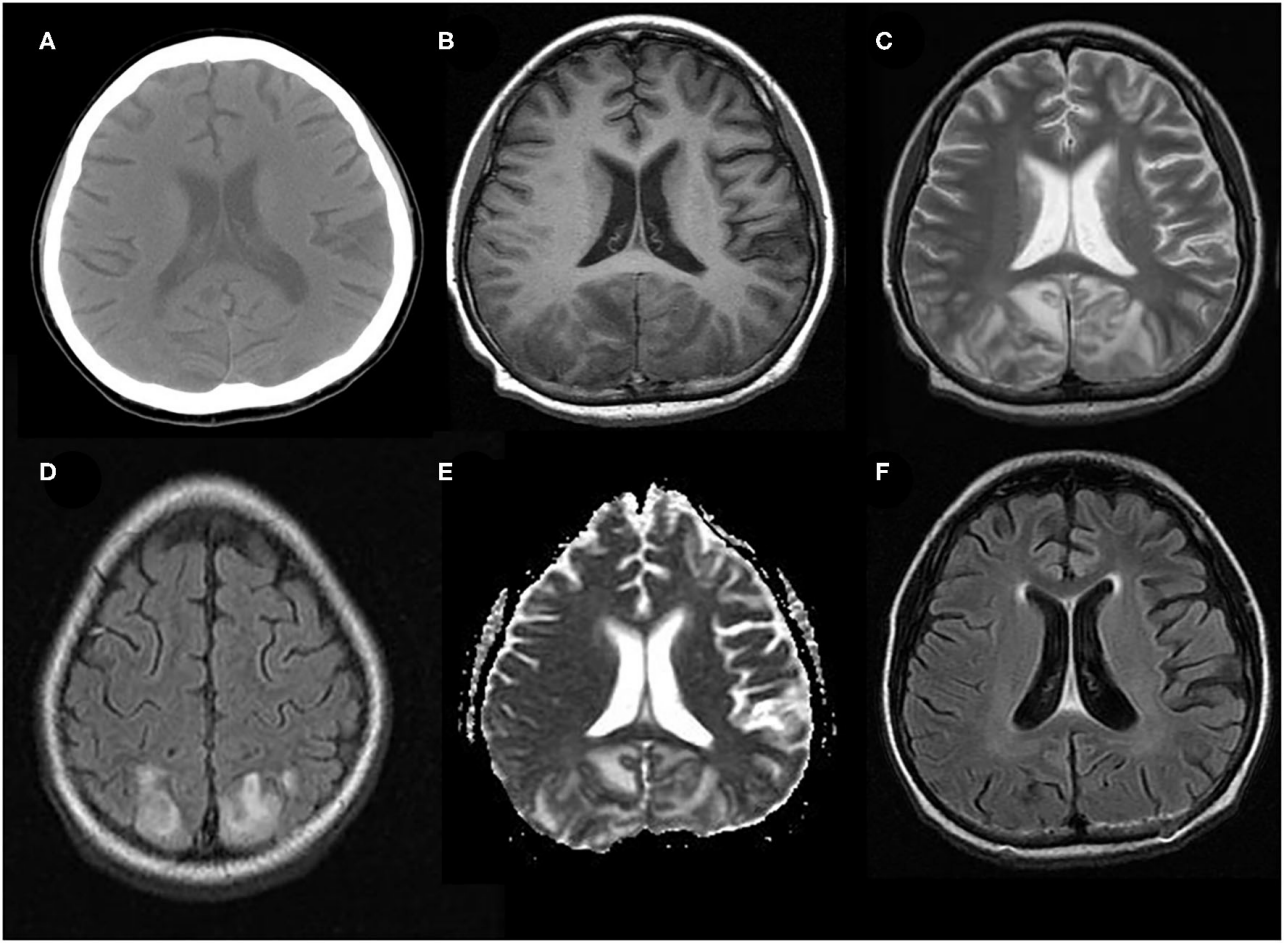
Typical neuroimaging of PRES in a 9-year-old male with underlying nephritic syndrome. (A) Initially, unenhanced brain CT showed mildly cortico-subcortical hypodensities, particularly in bilateral parieto-occipital regions. (B) At the acute stage, these cerebral lesions showed hypointensities on T1-weighted MRI in bilateral parieto-occipital regions (C) T2-weighted MRI, and (D) T2-FLAIR MRI showed symmetrical hyperintensities in bilateral parieto-occipital regions. (E) ADC map shows a hyperintense signal in the aforementioned corresponding areas. (F) FLAIR images 2 months later, showing a complete resolution of the abnormal signal.
